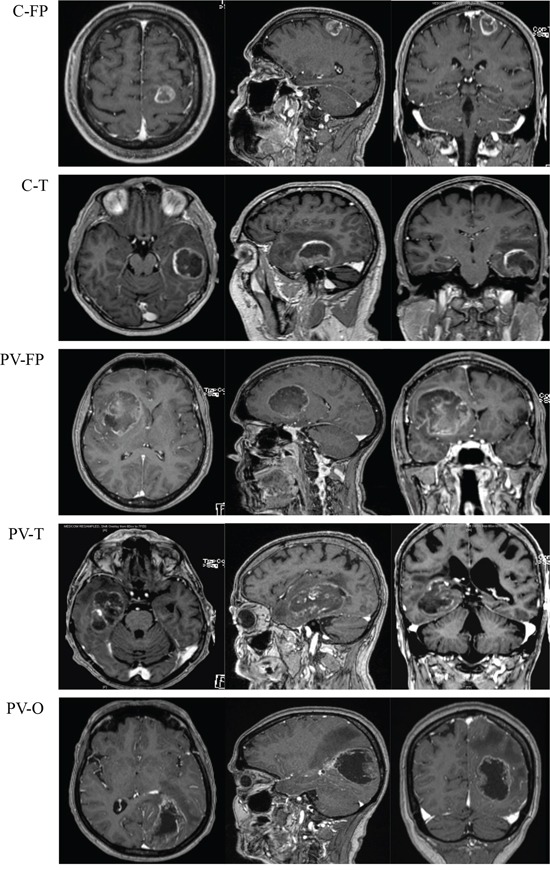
Magnetic resonance imaging with T1 enhancement (axial (left), sagittal (middle) and coronal (right)) of five tumor locations Abbreviations: Cortical fronto-parietal (C-FP), Cortical temporal (C-T), Periventricular fronto-parietal (PV-FP), Periventricular temporal (PV-T), Periventricular occipital (PV-O).
